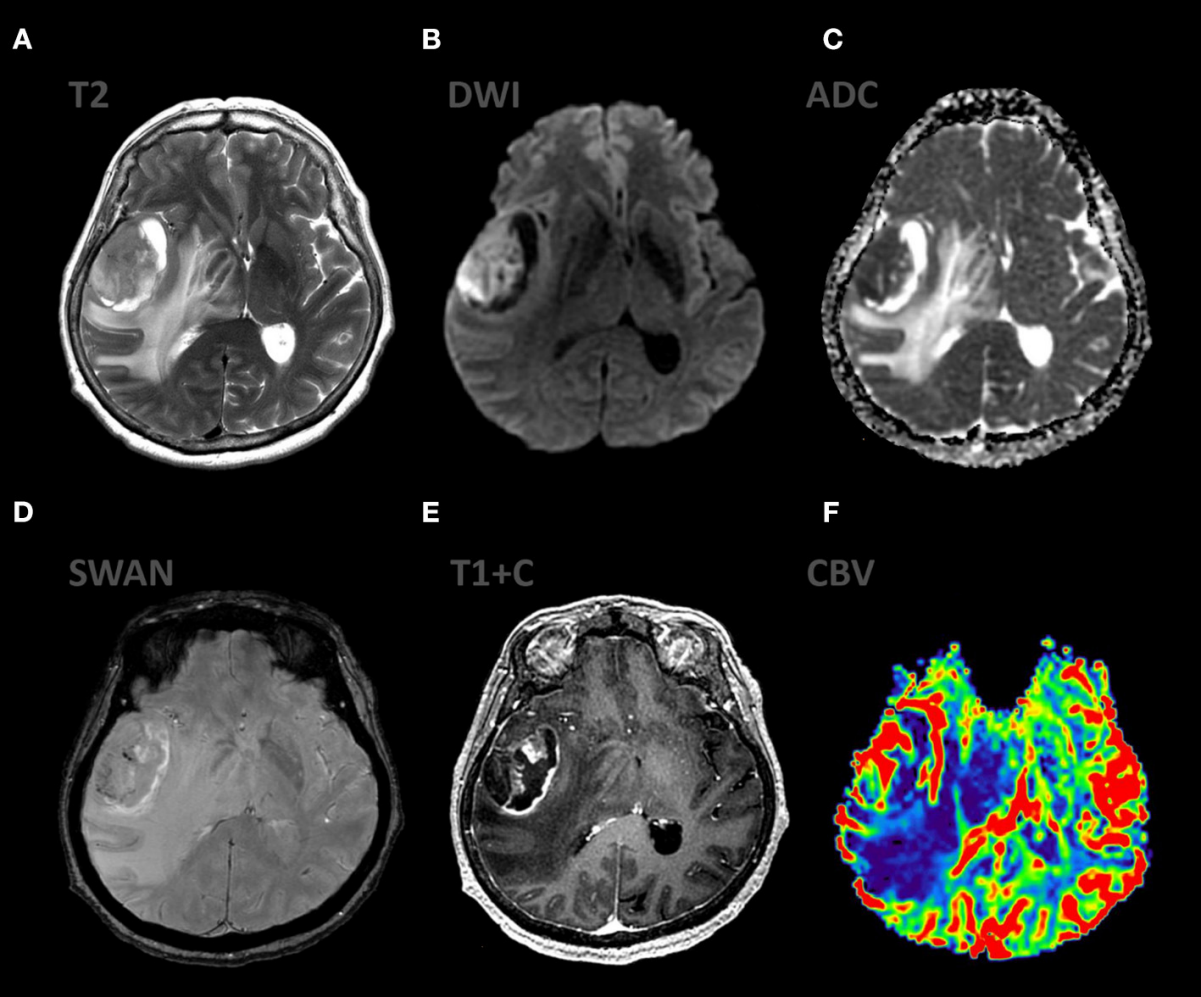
mpMRI ax: (A) T2WI; 6, (B) DWI, (C) ADC, (D) SWAN, (E) T1 + C, (F) DSC-PWI show the classic appereance of GBM (grade IV WHO 2016). The mass shows ring enhancing pattern associated with extensive peripheral T2-hyperintensity and moderate mass effect. The enhancing component of the lesion demonstrates restricted diffusion (ADC 800–900 × 10^−6^ mm^2^/s), also the mass shows high values rCBV.
